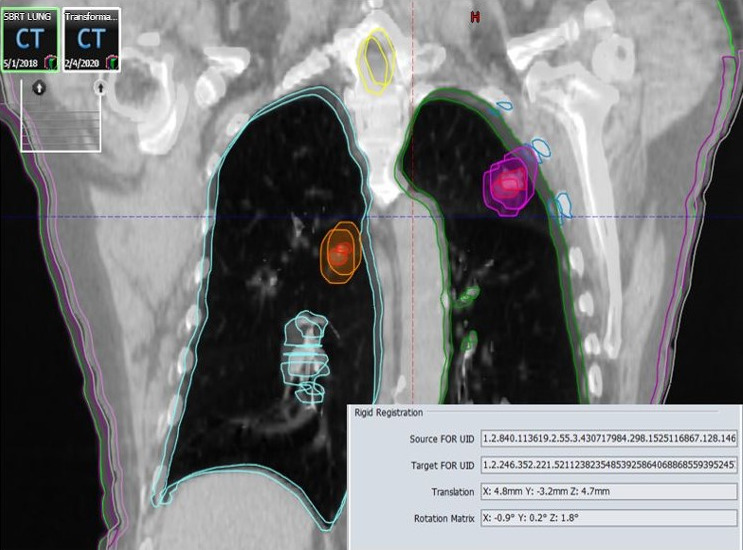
Above is a demonstration of a randomly rotated (within, ±2°) and translated (within, ±5 mm) CT data set (see bottom right inset) around the plan isocenter location (cross‐hair) for a representative patient (one fraction). The PTVs are shown in orange and pink and the GTVs are in red in both lungs. Normal structures are shown: lungs (light blue and green), skin (purple), cord (yellow), and ribs (blue). This patient was treated for 50 Gy in 5 fractions to both tumors, thus the random transformation process was repeated for a total of five treatments (see top left inset).
